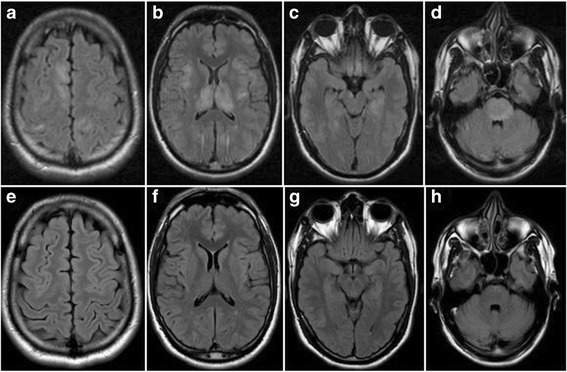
FLAIR sequence from the brain MRI at onset (a-d) and after treatment (e-h). Cortical and subcortical damage (a-b) with severe bilateral involvement of thalamus and internal capsule (b), mesial temporal lobes (c) and pons (d) in absence of contrast enhancement, is seen on brain MRI performed ad onset. After treatment with IVIg a significant improvement was noted with an almost complete resolution of the cortical, thalamic and basal ganglia involvement (e-f) and a reduction of temporal (g) and brainstem lesions (h)
